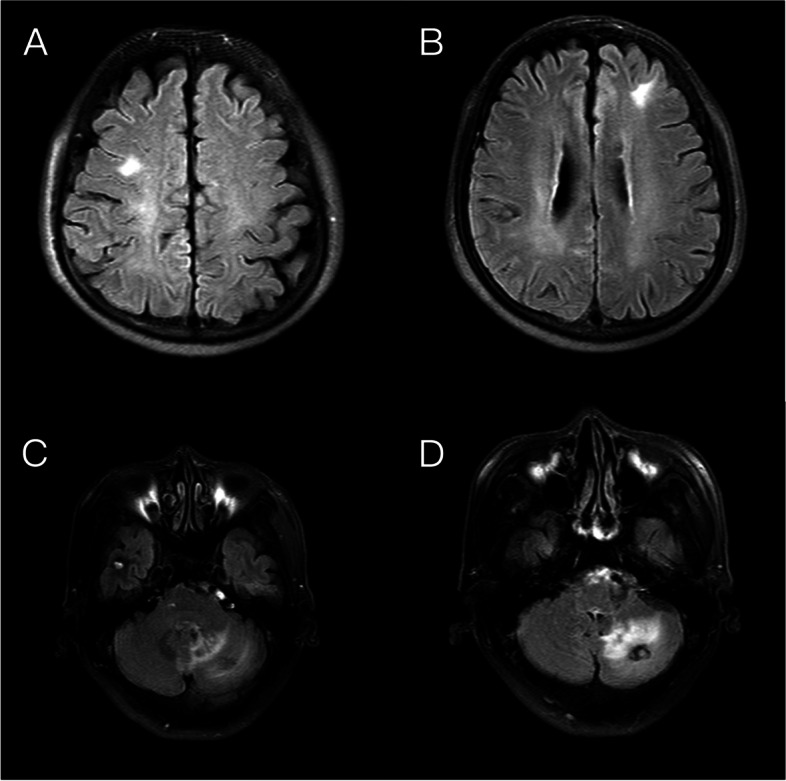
The brain MRI prior to tislelizumab administration in April 2021. Multiple metastasis lesions in the right frontal lobe (A), left frontal lobe (B), right temporal lobe (C), and left cerebellar hemisphere (D)
